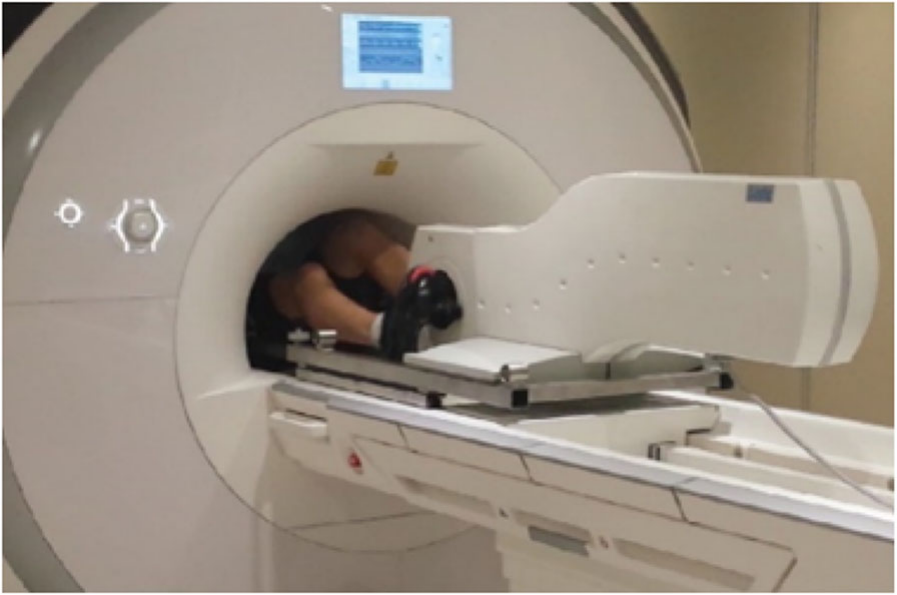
Exercise is performed within the MRI scanner (top image) using the Lode MR Ergometer Pedal with images acquired in real-time during exercise. Exercise is performed at workloads individualised from each participant’s peak workload from their upright cardiopulmonary exercise test
